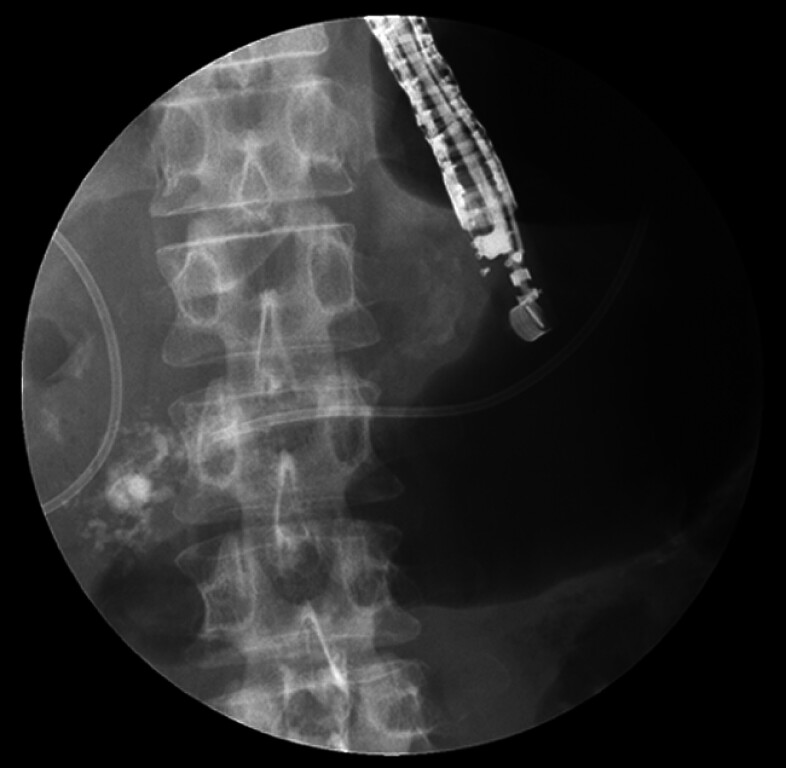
Placement of a plastic stent during initial endoscopic ultrasound-guided pancreatic duct drainage.
